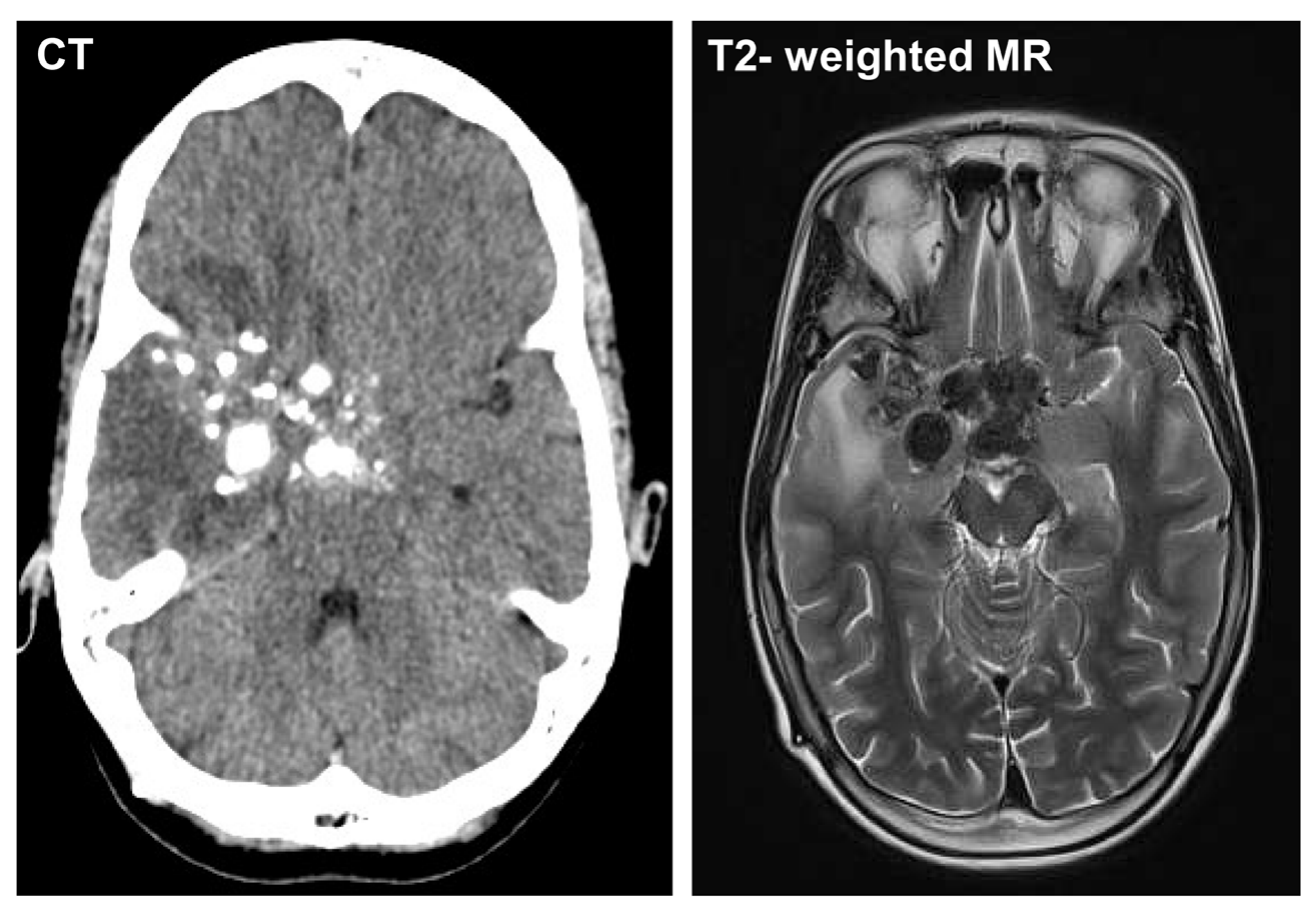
Corresponding magnetic resonance (MR) and computed tomography (CT) images of tuberculomas demonstrating calcification by CT imaging of lesions that appear hypointense (“T2-black”) by T2-weighted MRI.
